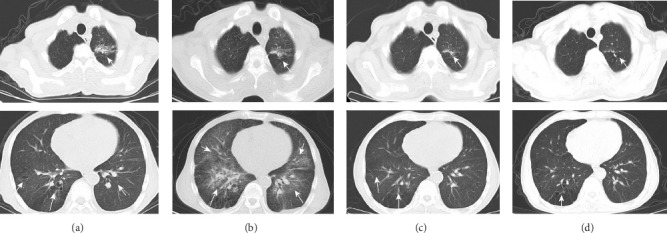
Serial chest computed tomography (CT) findings throughout the treatment course. (a) Chest CT image upon admission. (b) Chest CT showing progression of infection. (c) Chest CT demonstrating improvement following treatment. (d) Final CT at treatment completion revealing stable fibrotic changes without active disease. White arrows indicate lesions.
